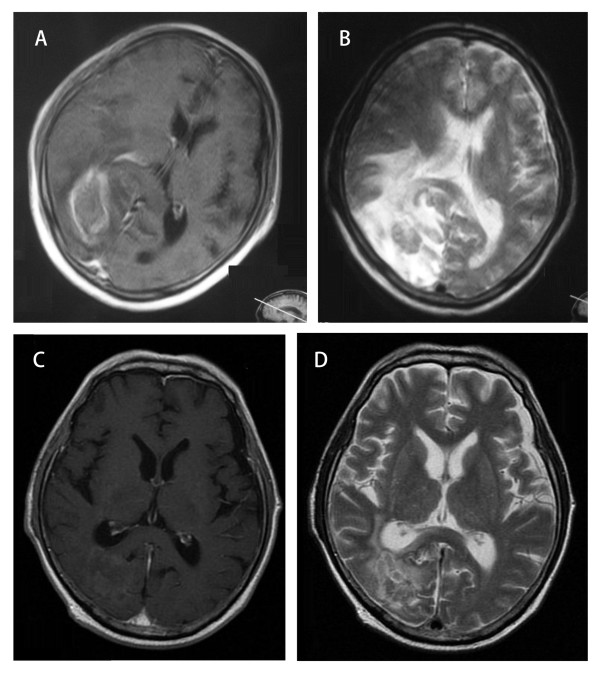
MRI images before (A, B) and after (C, D) bevacizumab treatment in Patient No. 5. Patient No. 5 (Table
